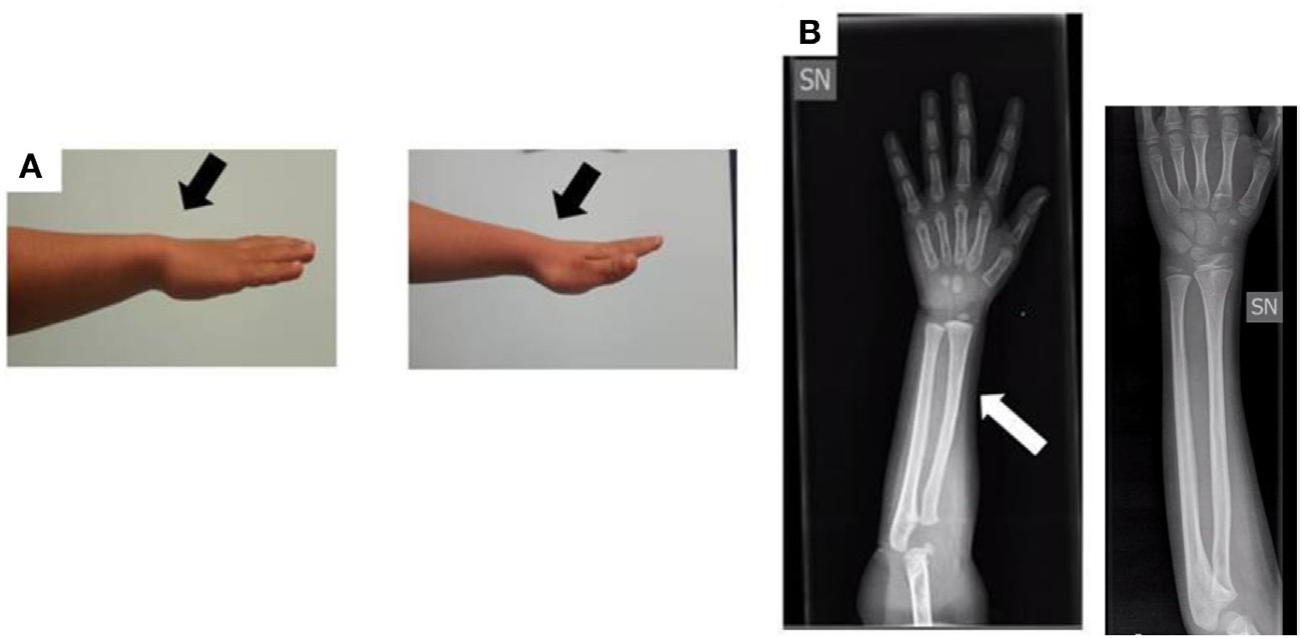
(A) Photograph of the forearm of the proband. No Madelung deformity is present (black arrows). (B) Radiograph of the forearm of the proband at the age of 3 years: the white arrow shows a mild bowing of the radius in comparison to a normal radiograph (the one without arrow).
